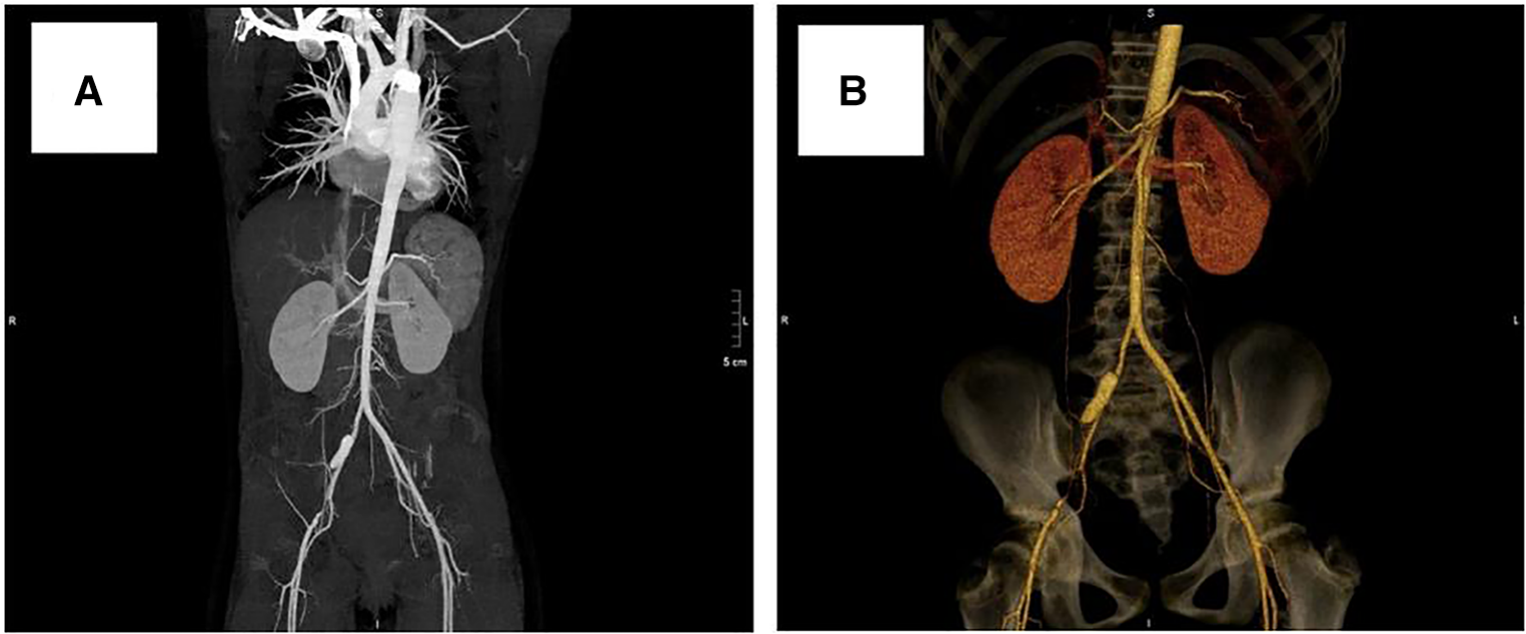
Computed tomography scan showing dissection of the right femoral artery: (A) 3 days after stenting, (B) 3 years after stenting.
